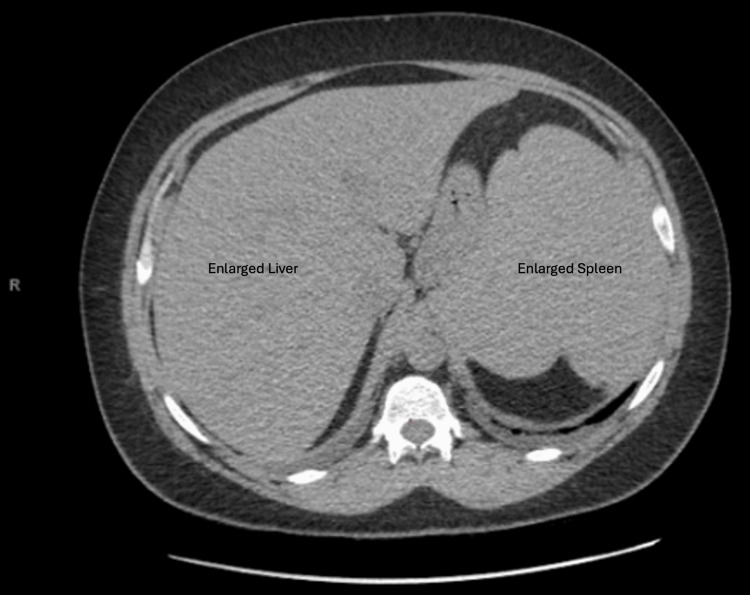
Axial view of the CT scan of the abdomen and pelvis shows hepatomegaly and splenomegaly.
